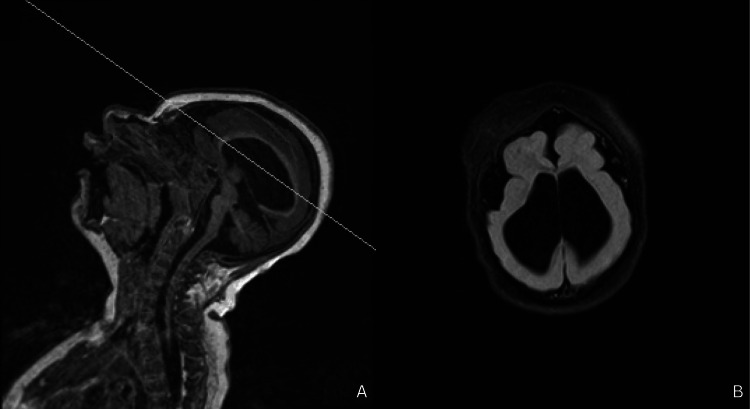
MRI brain (sagittal and axial view) A: On sagittal view, ventriculomegaly, brainstem hypoplasia, and cerebellar vermis hypoplasia can be appreciated; B: On axial view, there is a notably thin corpus callosum, lateral ventriculomegaly, and diffuse agyria that is more prominent posteriorly.
